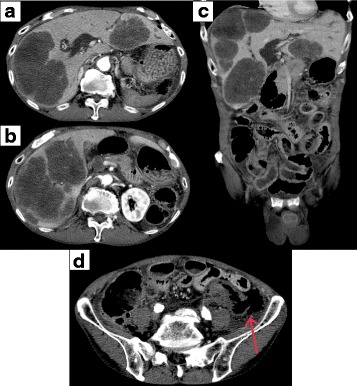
CT scan of the abdomen. a–c Multiple liver metastases were revealed. d Abdominal free air and perforating sigmoid colon cancer (arrow) were revealed
